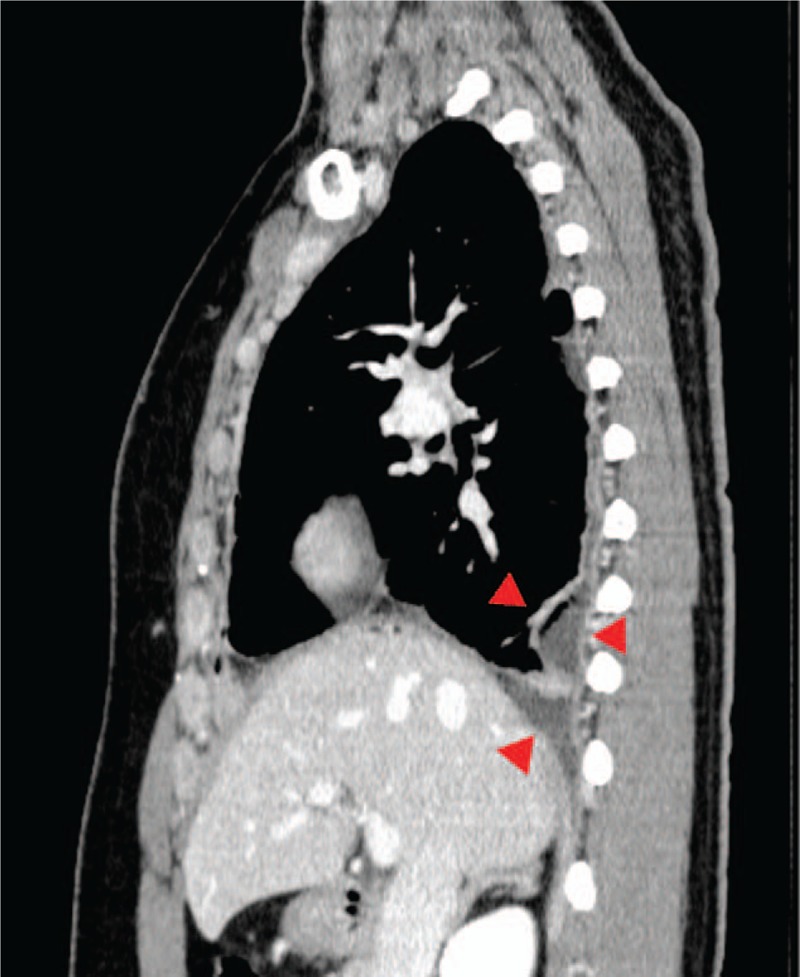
The contrast-enhanced chest CT image shows thickening of the right pleura and residual pleural effusion (arrowheads) on day 6 postadmission.
